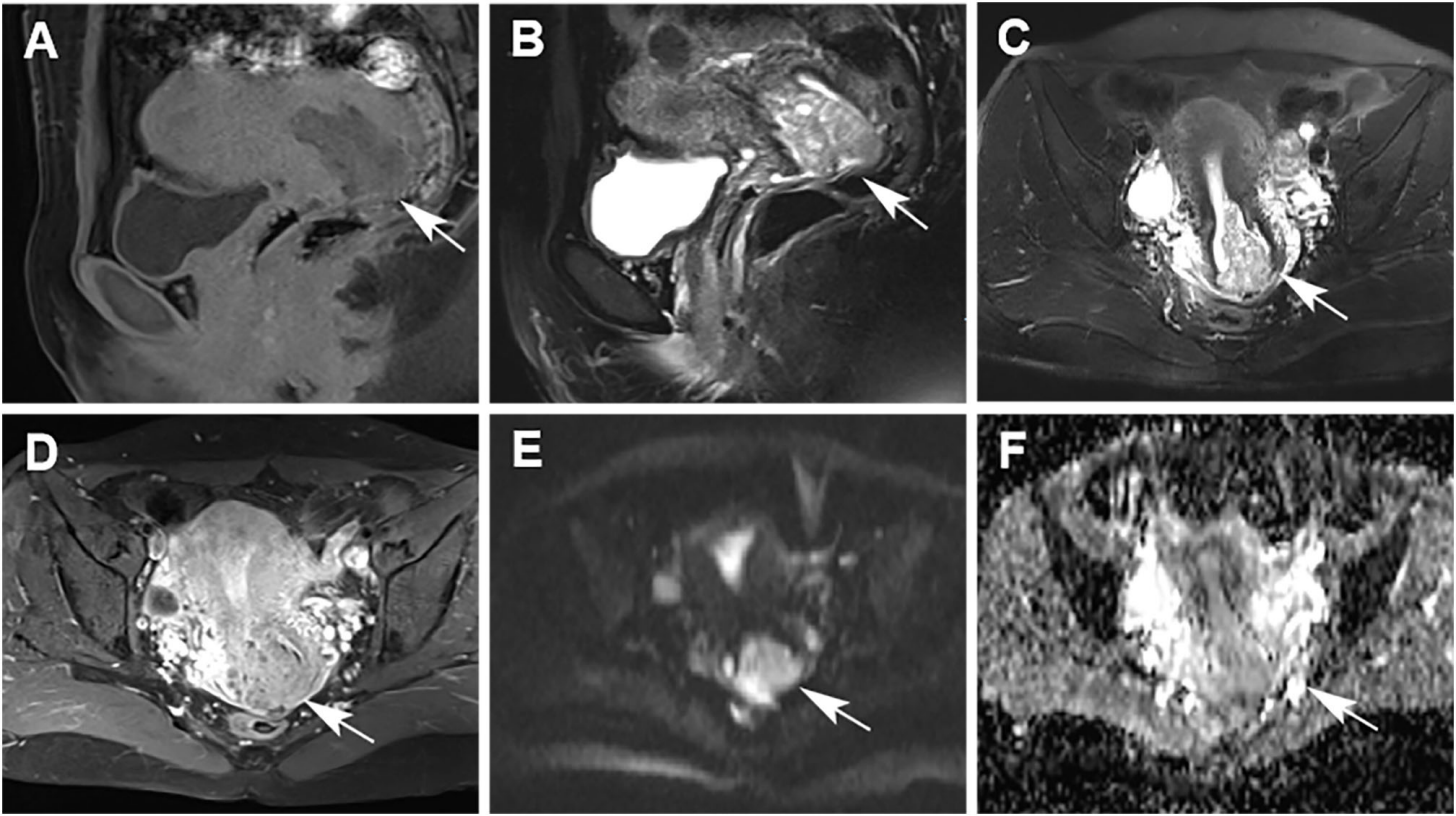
Magnetic resonance imaging (MRI) revealed a mass-like soft tissue tumor in the cervix. (A) MRI showed a slightly low signal on T1-weighted imaging (white arrow). (B) MRI showed a high signal on T2-weighted sagittal imaging (white arrow). (C) MRI showed a high signal on T2-weighted axial imaging (white arrow). (D) Contrast-enhanced magnetic resonance imaging (CE-MRI) showed that the lesion was significantly enhanced on contrast-enhanced scanning of T1-weighted imaging (white arrow). (E) High signal on diffusion-weighted imaging (DWI) (white arrow). (F) Reduced signal on apparent diffusion coefficient (ADC) (white arrow).
